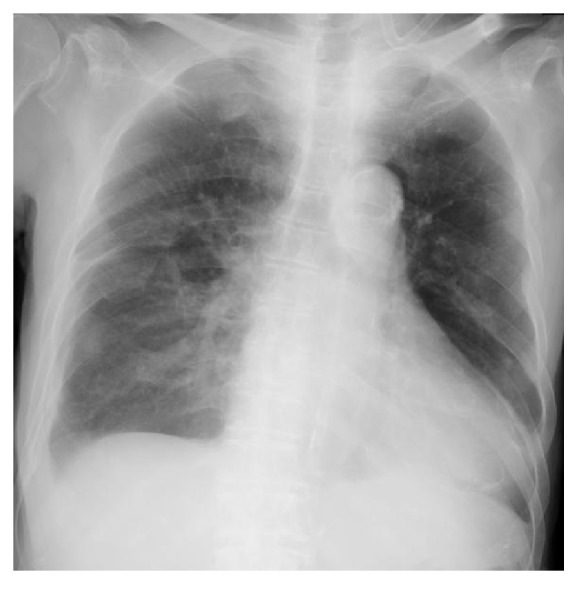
Chest radiography on admission revealed mild widening of cardiac silhouette and right pleural effusion.
